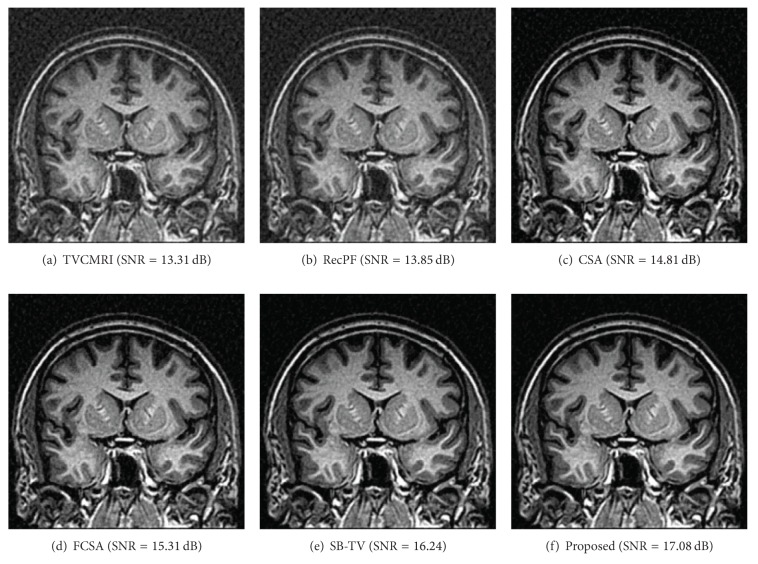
Brain MR image reconstruction from 20% samples (Rician noise case).
